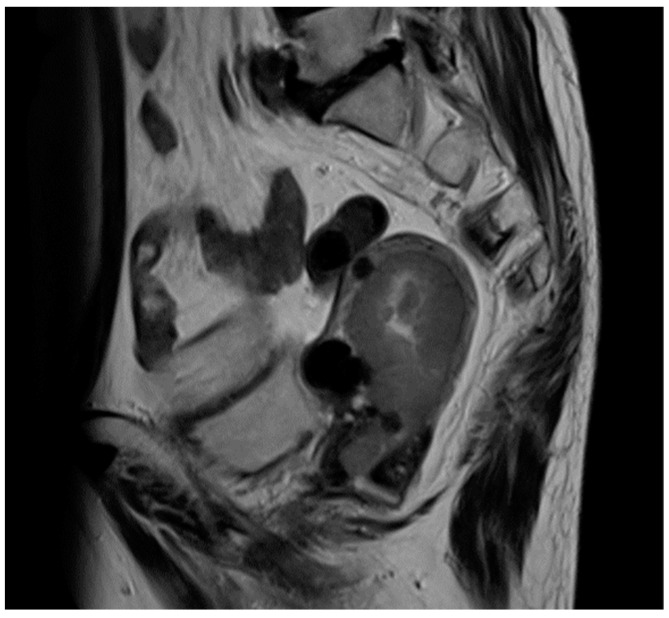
Magnetic resonance imaging of the pelvis. T2-weighted imaging revealed a thickened endometrial mass (50 × 73 × 43 mm).
